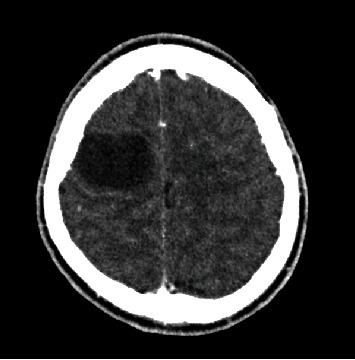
Axial postcontrast CT scan image showing rim enhancing hypoattenuating lesion in the right frontal lobe suggestive of abscess.
